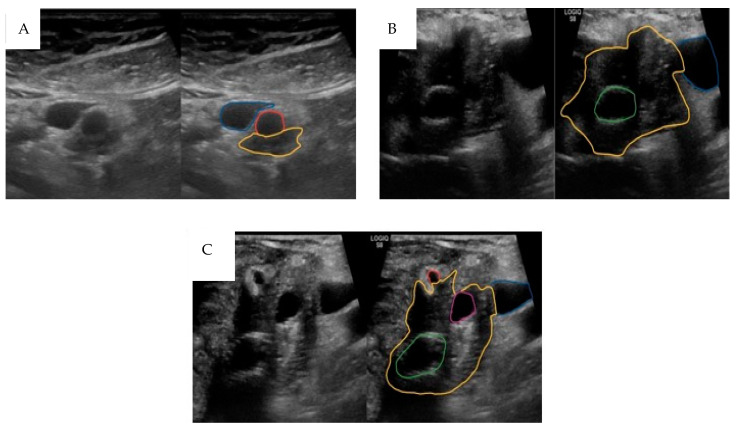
Representative non-annotated and annotated clinical images of ultrasound imaging of pancreatic ductal adenocarcinoma (PDAC). (A) Preoperative transabdominal ultrasound of pancreatic tumour extension (yellow) posterior to the 90–180 degrees affected superior mesenteric artery (unaffected wall marked red) and close to the superior mesenteric vein (blue, unaffected). (B) Intra-operative ultrasound of PDAC (yellow), with common bile duct stent (green) and <90 degrees affected superior mesenteric vein (unaffected wall in blue). (C) Intra-operative ultrasound of PDAC (yellow), with common bile duct stent (green) and 90 degrees affected superior mesenteric vein (unaffected wall in blue), 90 degrees affected gastroduodenal artery (unaffected wall in red), and dilated pancreatic duct (in purple). Data are original and unpublished.
